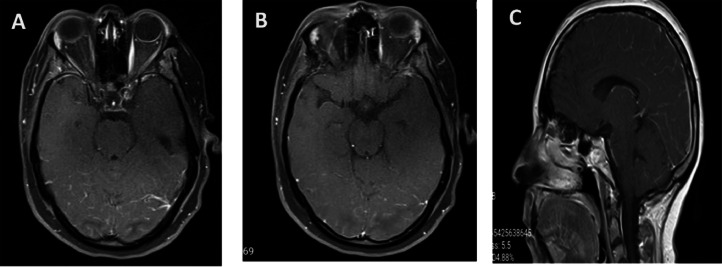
Brain MRI axial (A and B) and sagittal (C) Postcontrast images show leptomeningeal enhancement in parieto-occipital lobes, compatible with PRES.
